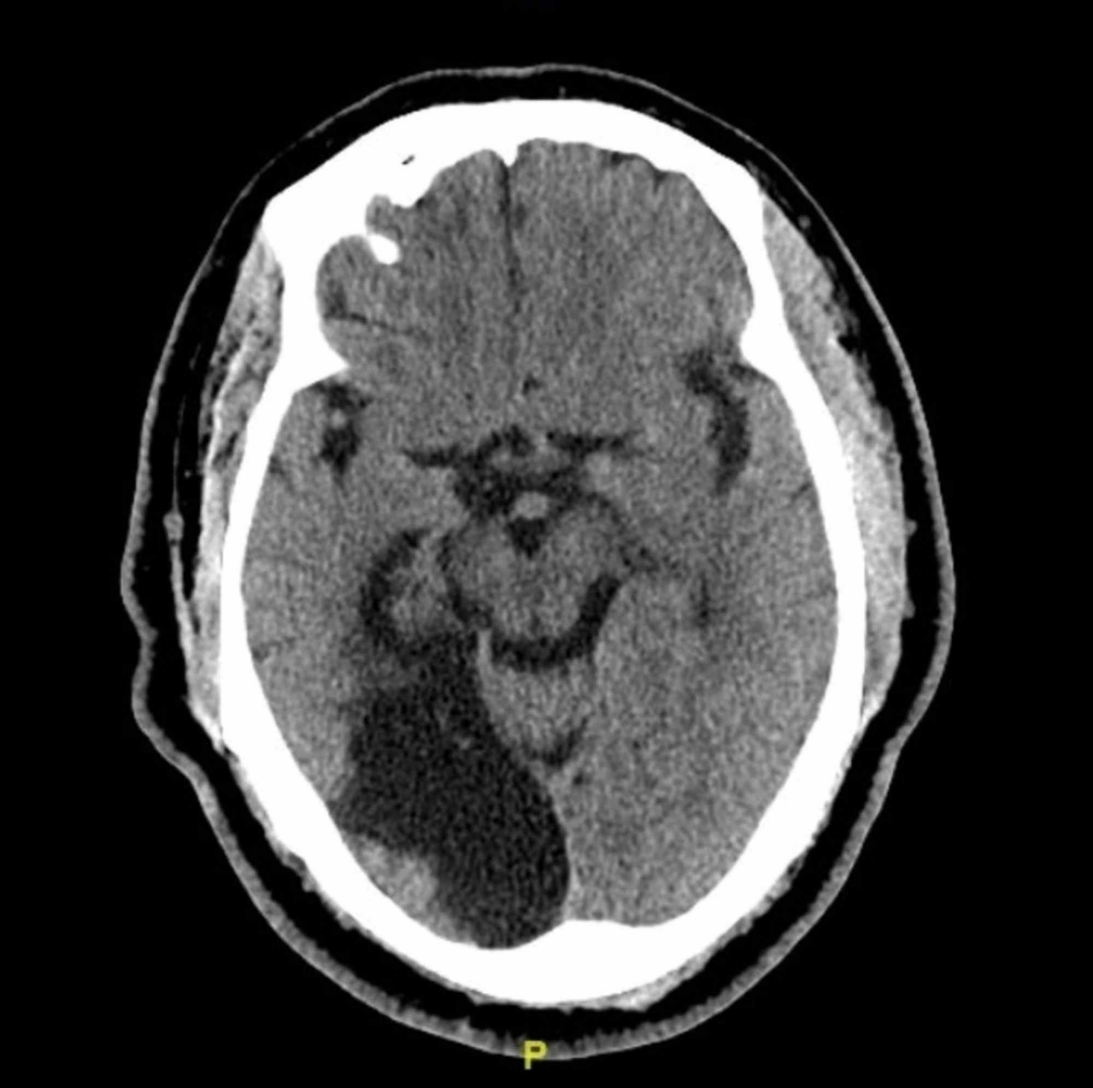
CT scan of brain without contrast Old right posterior cerebral artery territorial infarct involving the occipital and temporal lobes
